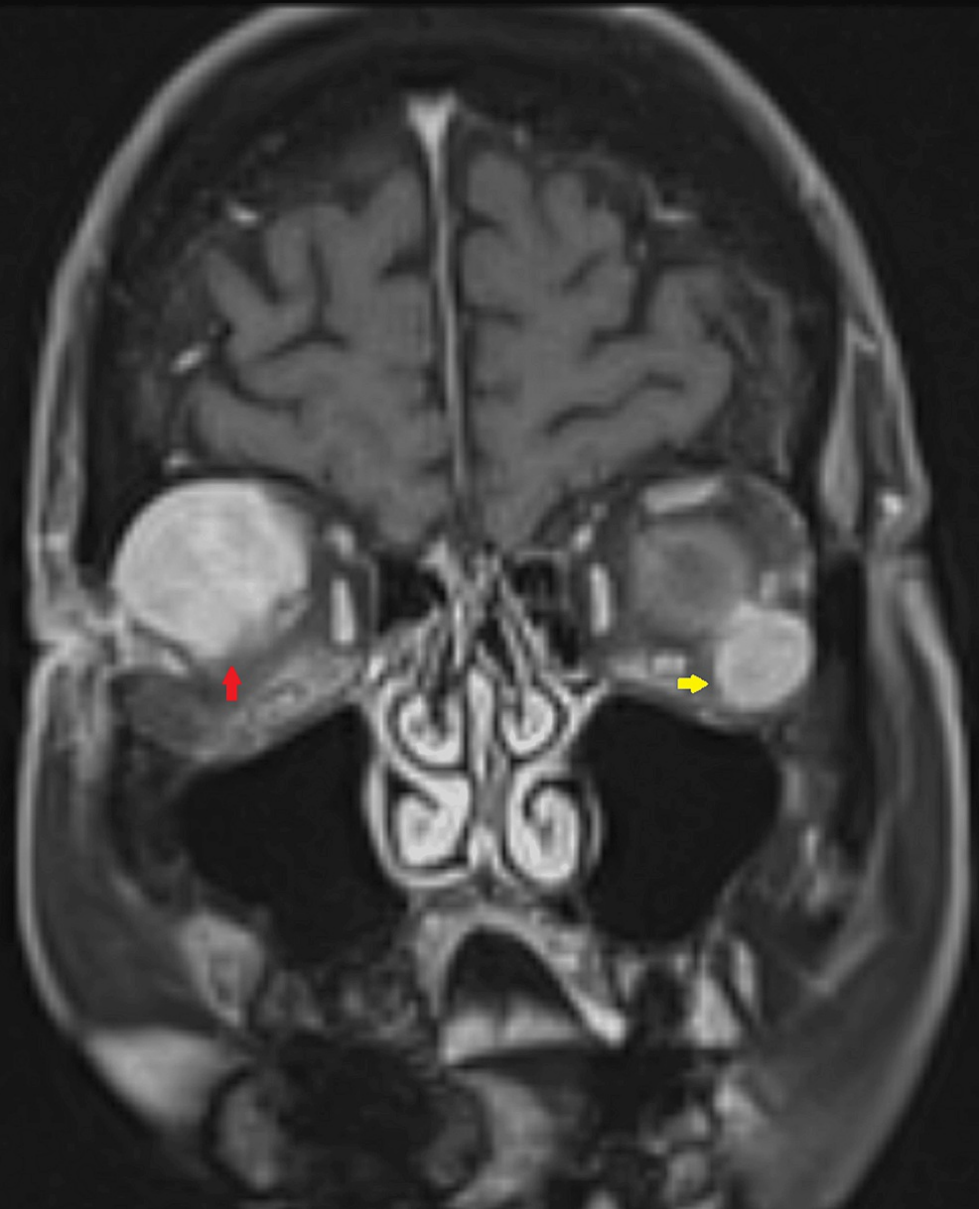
T1 post-contrast brain MRI image (coronal view) Uniformly enhancing, bilateral intra-orbital lesions are seen (red and yellow arrows). The lesions are closely abutting the adjacent extraocular muscles. Note the larger lesion in the right orbit encasing the optic nerve and compressing and displacing it inferiorly.
